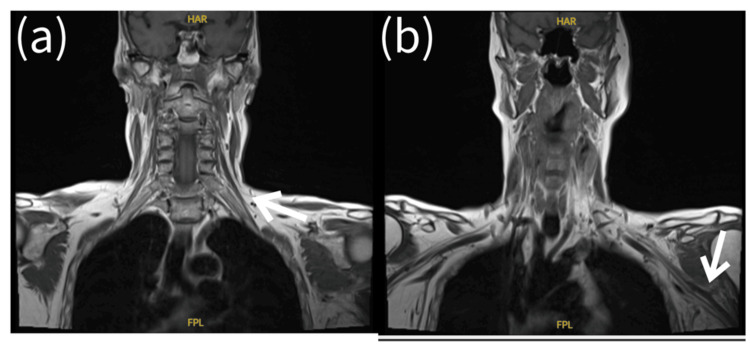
MRI brachial plexus findings (a) Demonstrates preserved nerve continuity in the left proximal brachial plexus. (b) Demonstrates edema around the left distal brachial plexus but preserved nerve continuity.
